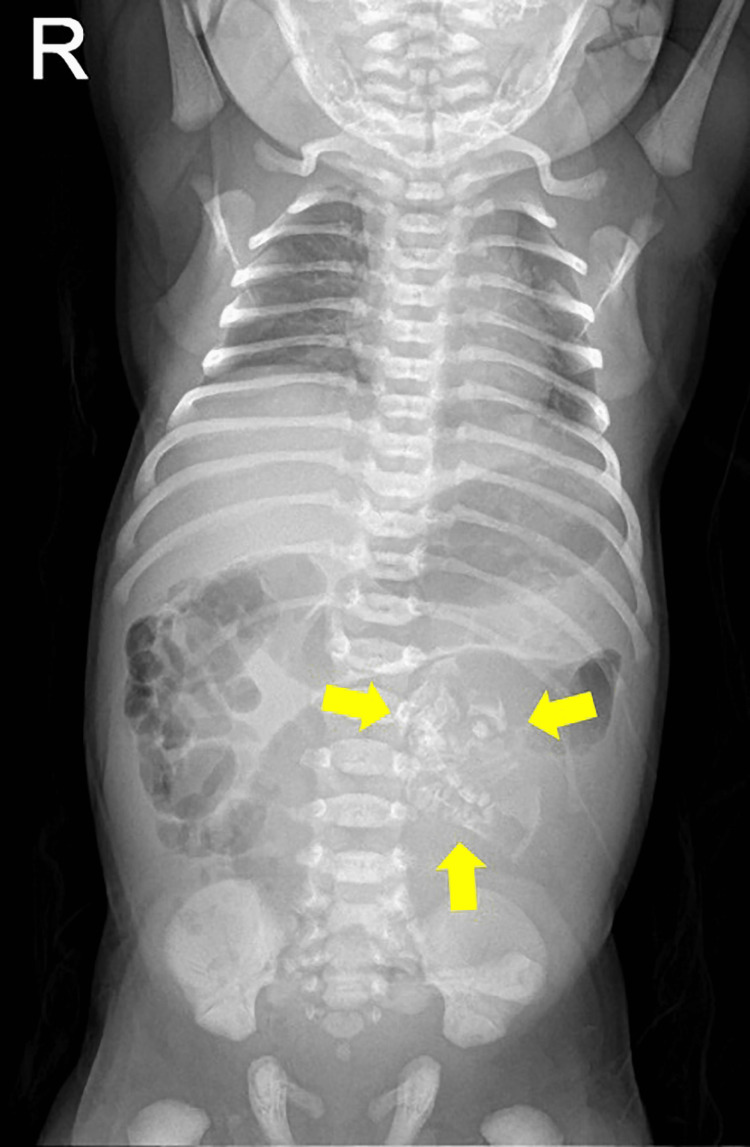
Plain baby-gram X-Ray showing multiple calcifications in the left abdominal area at the level of the L1-L4 vertebrae adjacent to the midline (yellow arrows).
